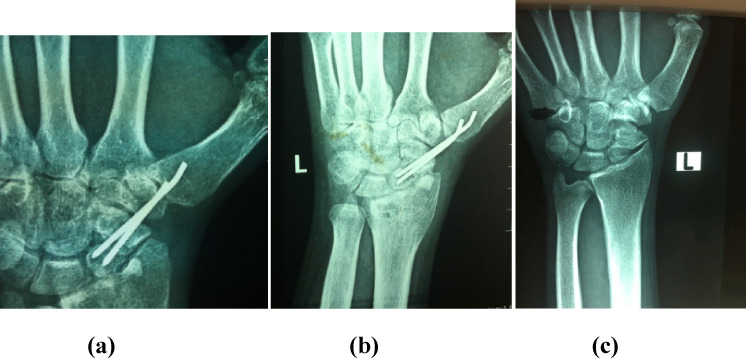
a) Postoperative PA wrist X-ray of revision fixation of non-united scaphoid using k wires and vascularized MFC graft. b) Follow-up X-ray. c) X-ray after wires removal showing full scaphoid union.
